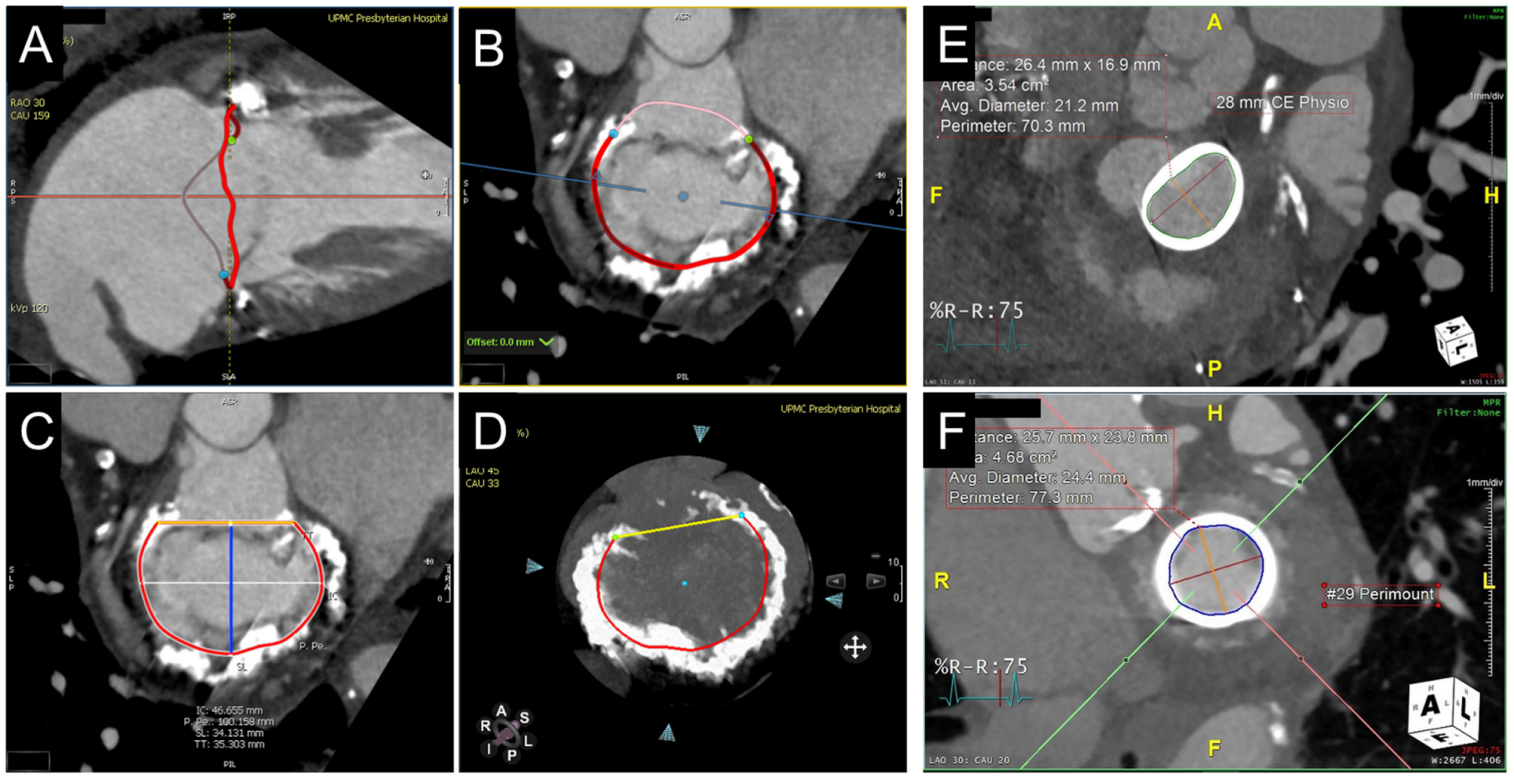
CT annular segmentation for TMVR. (A–D) Segmentation of the native mitral annulus for the planning of valve-in-MAC TMVR. As shown from a long-axis view (A), the mitral annulus outlined in red (A) has a non-planar saddle geometry. Projected into a short-axis view (B), the anterior horn (*pink border*) may be truncated to assume a D-shaped configuration. (C) The trigone-trigone (*orange*), septal-lateral (*blue*), and inter-commissural (*white*) distances are measured from this view. Maximum intensity projection (D) allows for assessment of the distribution and density of annular calcification. (E) Valve-in-ring TMVR measurements of a complete semi-rigid ring with the major diameter in the inter-commissural dimension (*red*) and the minor diameter in the septal-lateral dimension (*orange*). (F) Valve-in-valve TMVR assessment showing the internal diameter slightly under the manufacturer specifications for the bioprosthesis.
